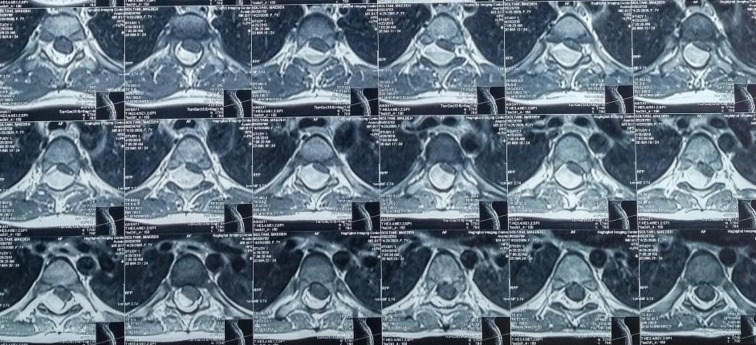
Axial T1 weighted MRI showed spinal subdural hematoma with compression effect to spinal cord (Arrow)
